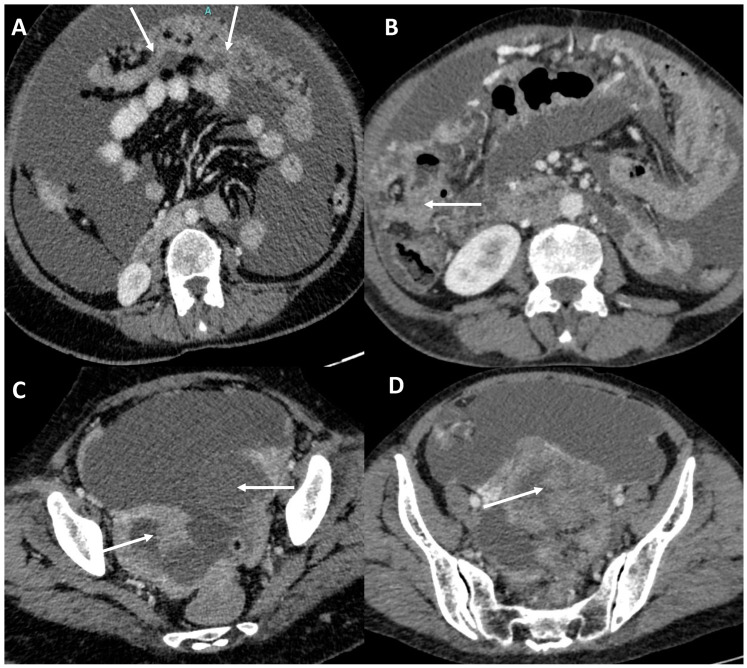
A 54-year-old female with peritoneal carcinomatosis. Axial contrast enhanced CT images show gross ascites with marked omental soft tissue thickening (“omental caking”, arrows, (A)) and serosal deposit along the ascending colon (arrow, (B)). Also note, bilateral adnexal masses (arrows, (C)) and large pelvic soft tissue deposit (arrow, (D)).
